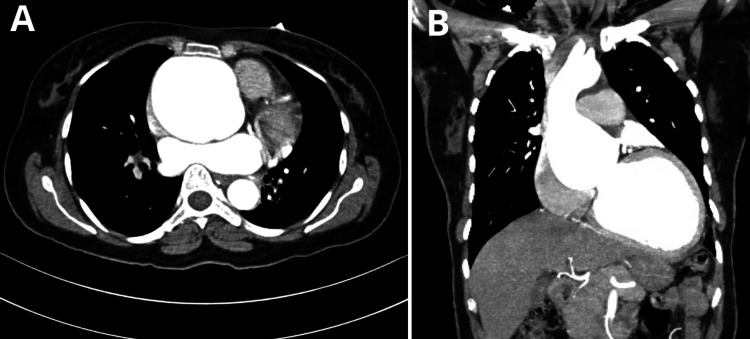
CT angiogram showing (A) axial view with a dilated ascending aorta and (B) coronal view displaying a dilated aortic annulus (2.7 cm) along with a proximal ascending aortic aneurysm (7 cm). CT: computed tomography
